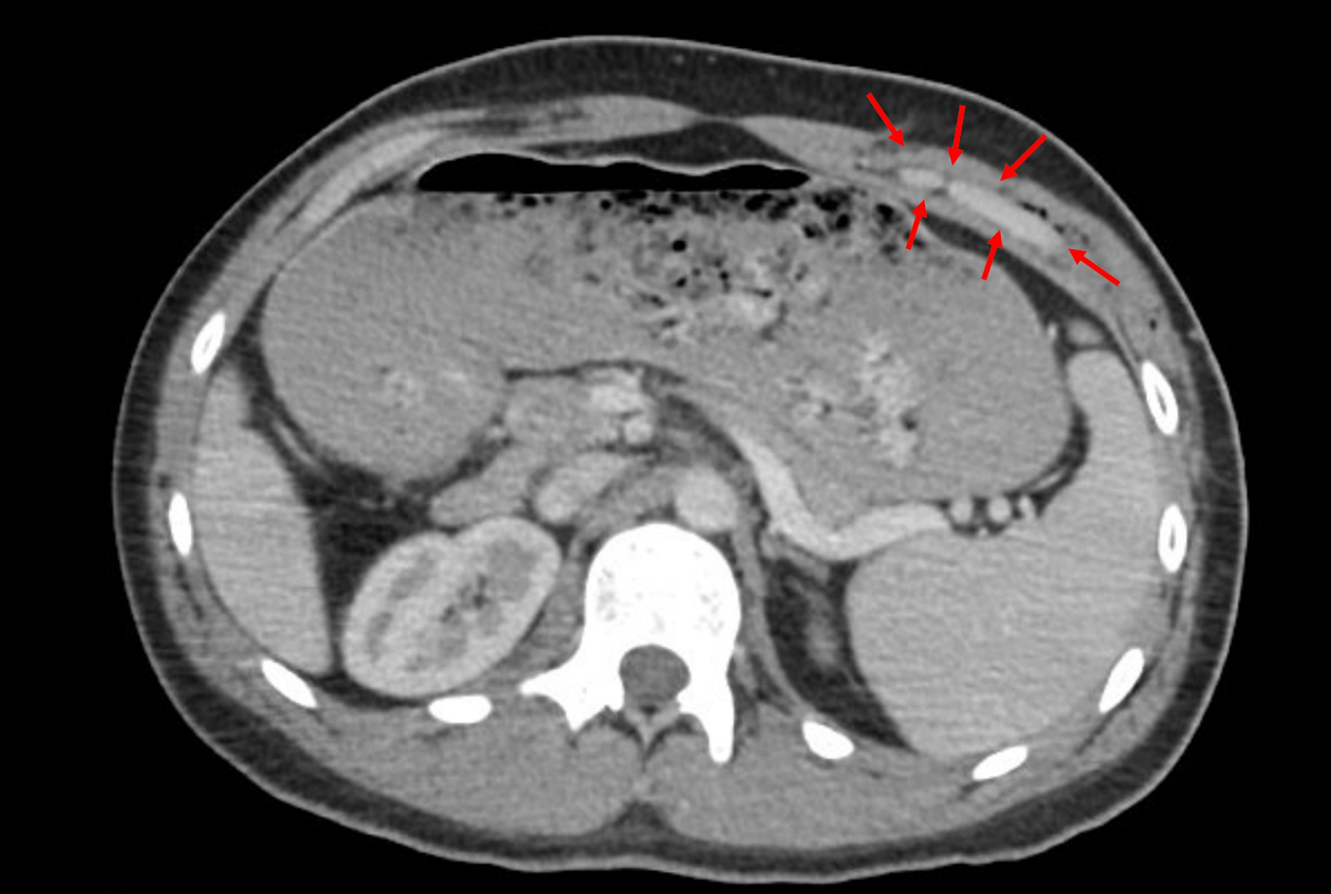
Initial ED CT with contrast revealed no evidence of a diaphragm or visceral injury. An upper left anterolateral abdominal wall hematoma is marked with red arrows, in axial view.
